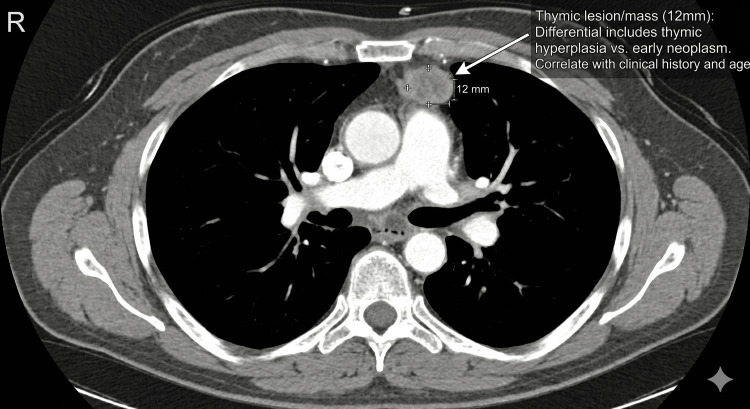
Chest computed tomography scan showing thymic hyperplasia in the anterior mediastinum Contrast-enhanced computed tomography (portal venous phase, slice thickness 2.5 mm) of the chest demonstrates diffuse, symmetric enlargement of the thymus measuring 12 mm in maximum thickness, without discrete nodules or invasive features.
